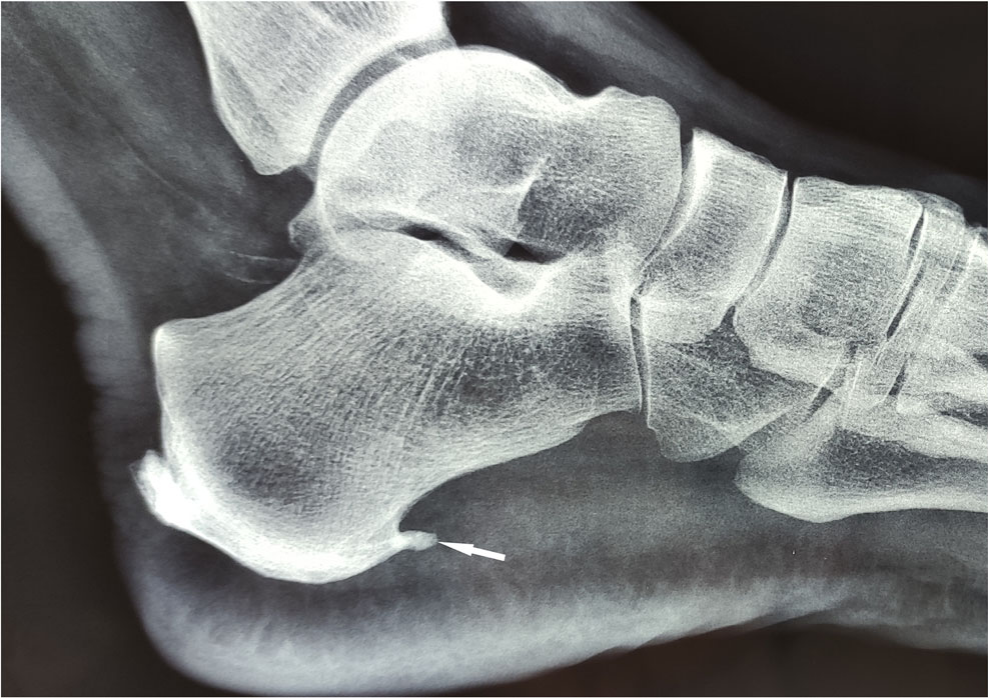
Plain radiograph of the left calcaneus. Arrow indicates plantar heel spur
